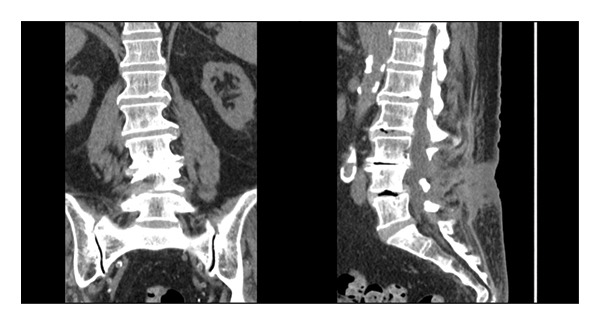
Computed tomography (CT) of the lumbar spine without intravenous contrast, sagittal and coronal reconstructions, demonstrating no prevertebral or paraspinal soft tissue edema to suggest discitis, osteomyelitis, or septic arthritis.
